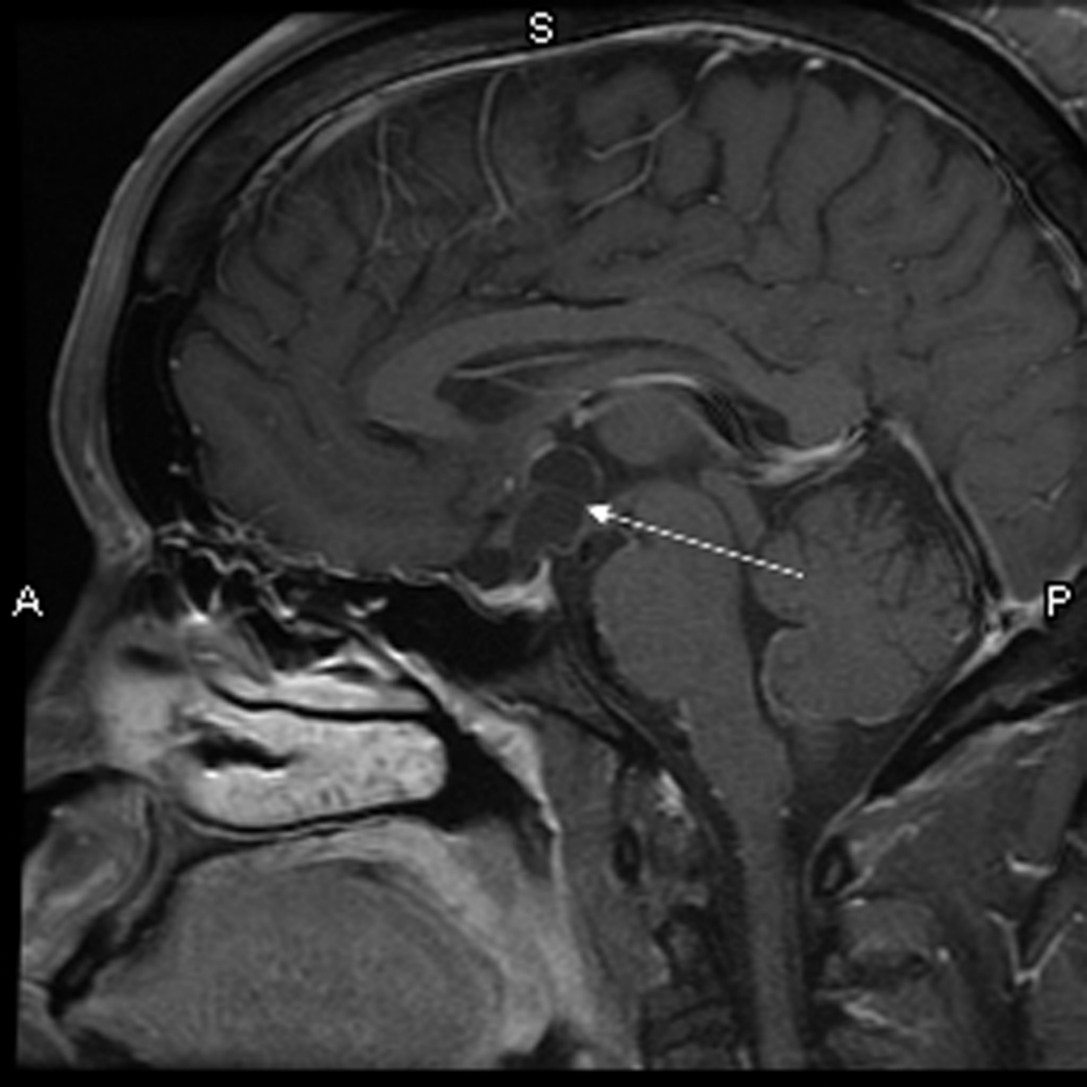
Sagittal T1 with contrast Preoperative brain MRI showing a multi-locular suprasellar cyst (solid white arrow) measuring approximately 1.6 x 1.8 x 2.4 cm
